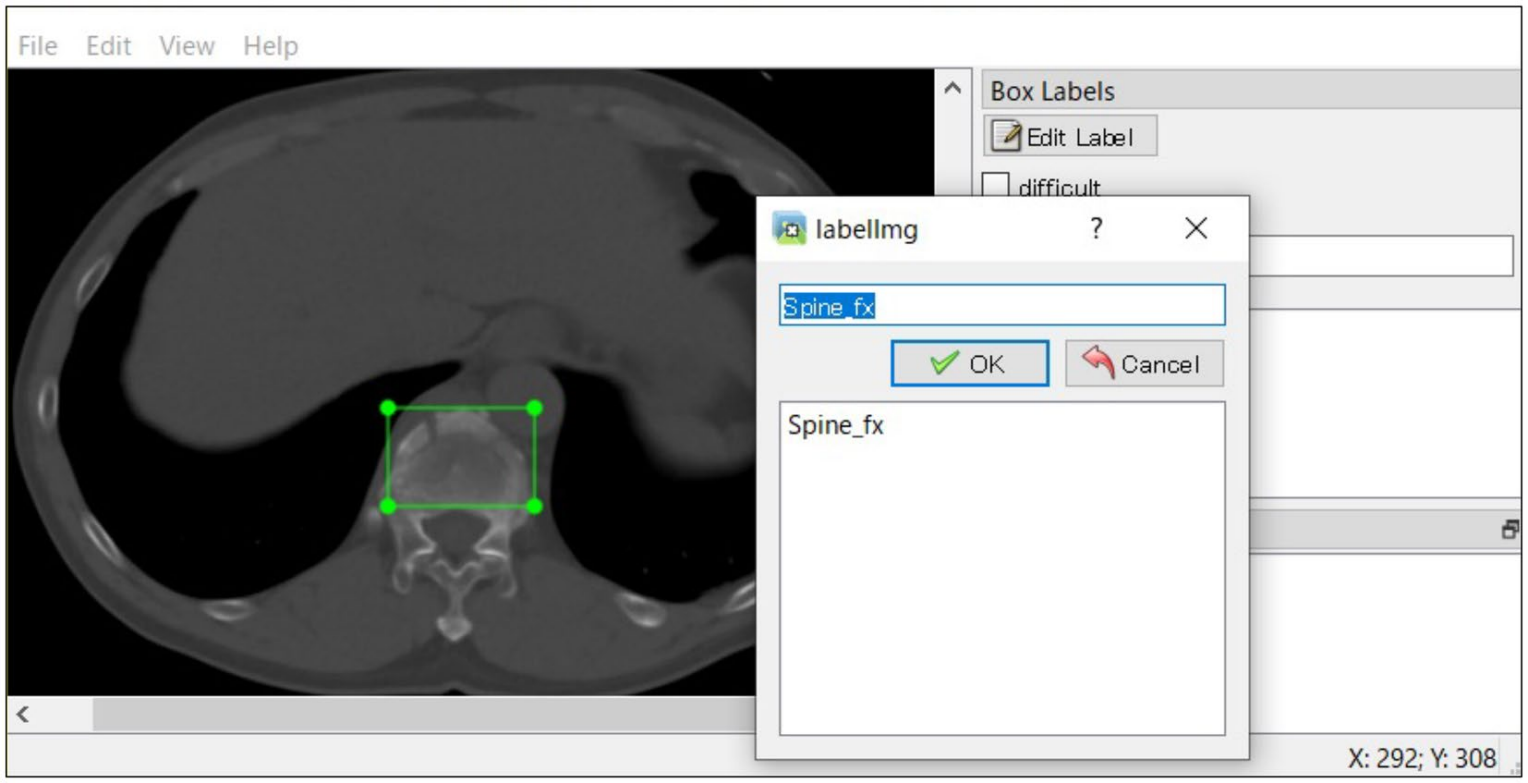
A screenshot of the image annotation process. We removed the black background and trimmed to include the area of the patient's trunk. A rectangular bounding box covering the minimum area of the fracture site was then drawn on every fracture of the CT slice and was labeled as spine fractures, pelvic fractures, or rib fractures using labelImg (version: 1.8.1, available at https://github.com/tzutalin/labelImg). CT, computed tomography.
